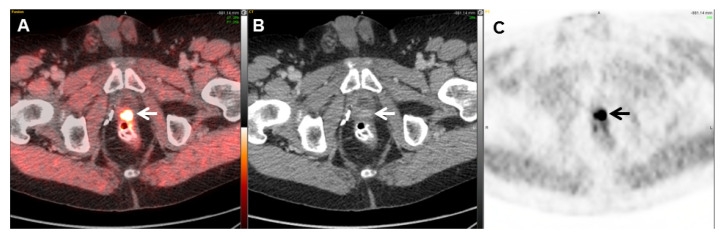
A 71-year-old man with prostate adenocarcinoma status post radical prostatectomy who had biochemical recurrence (PSA = 2.2 ng/mL). Axial fused PET/CT (A), CT (B), and PET (C) images demonstrate focal increased ^11^C-choline at the prostate bed (maximum standardized uptake value [SUVmax] = 5.0), compatible with tumor recurrence (arrows). The low urinary excretion of ^11^C-choline facilitates the evaluation of the prostatectomy bed.
